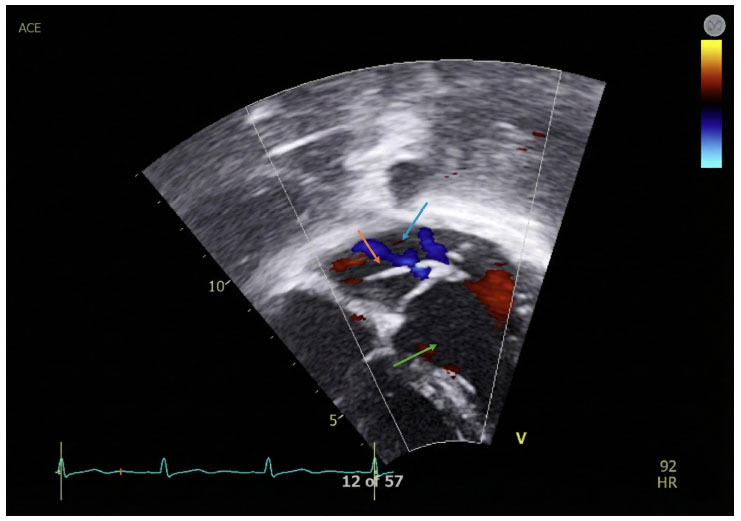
A transesophageal 2D echo with doppler with apical four-chamber view showing false tendon (orange arrow) during systole traversing the mitral valve from left atrium (blue arrow) to left ventricle (green arrow).
